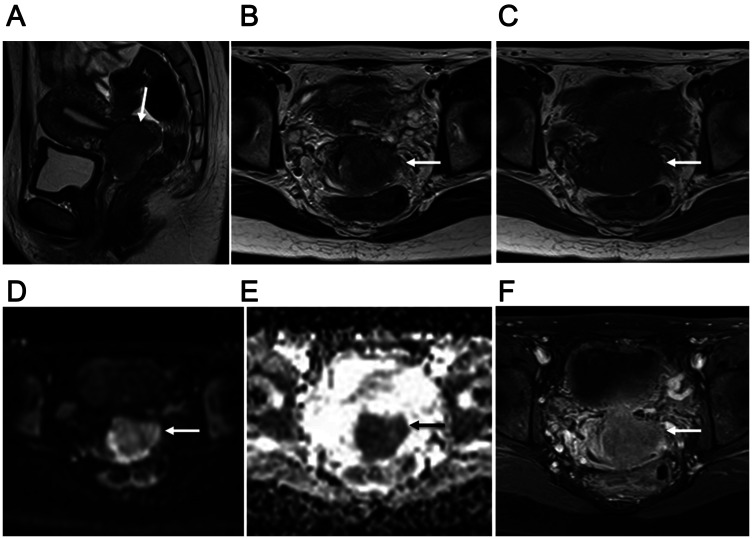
34-year-old woman with FIGO stage IIB2 uterine cervical small cell neuroendocrine carcinoma. (A) Lobulated mass with homogeneous and slightly hyperintense signal in the uterine cervix (arrow) shown by sagittal T2-weighted imaging. (B) Ill-defined mass with invasion of left parametrium (arrow) in axial T2-weighted image. Subsequent surgery findings confirmed that MRI staging of parametrium invasion was correct. (C) Iso-intense mass invading left parametrium (arrow) shown by axial T1-weighted imaging. (D) Cervical mass with remarkably high signal intensity (arrow) shown by axial DWI. (E) Obvious diffusion restriction throughout tumor (arrow) shown by axial ADC map. (F) Weak tumor enhancement (arrow) shown by axial late-phase enhanced fat-suppressed T1-weighted imaging (240 seconds after Gd-DTPA administration). Recurrence was noted 10.8 months after surgery and death at 37.2 months.
